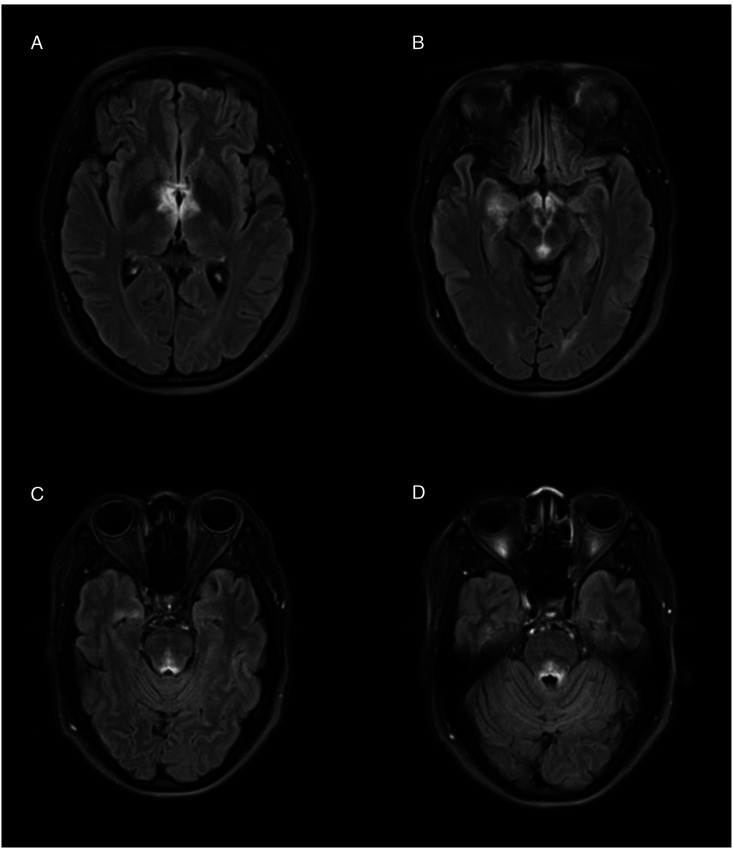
Patient’s initial T2 FLAIR MR brain imaging findings. (A) Involvement of the bilateral medial thalami and area surrounding the third ventricle. (B) Involvement of the bilateral mammillary bodies, periaqueductal grey matter and right medial temporal lobe. (C, D) Longitudinal extension with involvement of the fourth ventricle.
